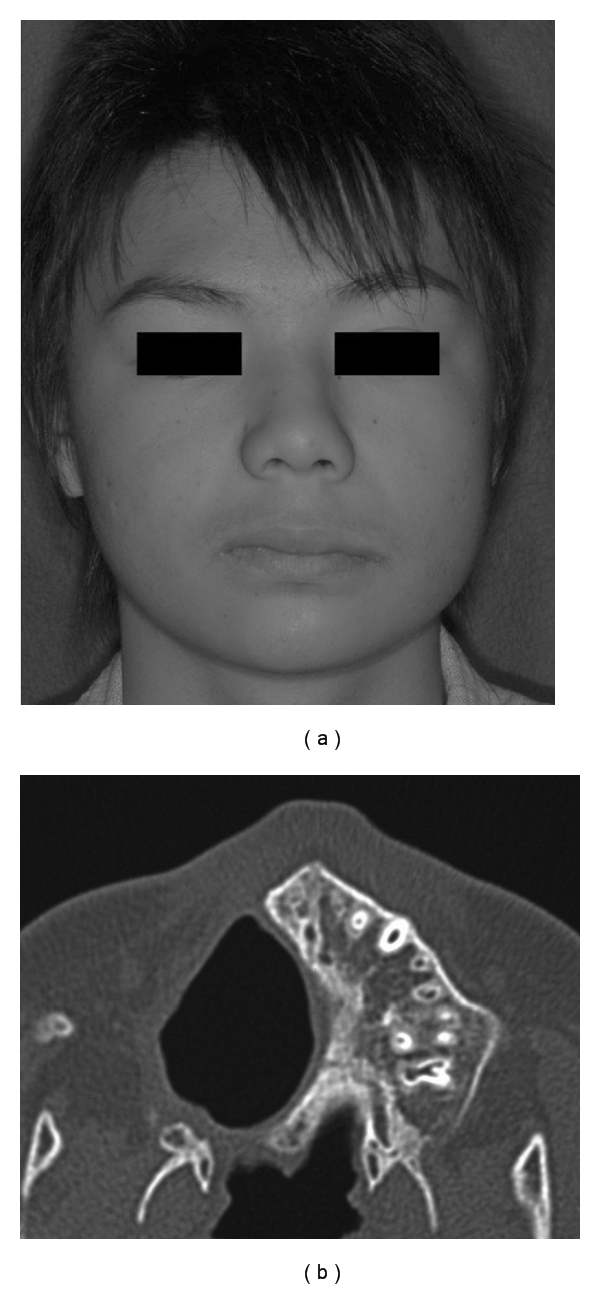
(a) Nine years after surgery, the patient has a normal appearance although the right corner of the mouth is slightly higher than the left. (b) CT taken at 9-year followup, showing the defect in the maxilla and no recurrence of tumour.
